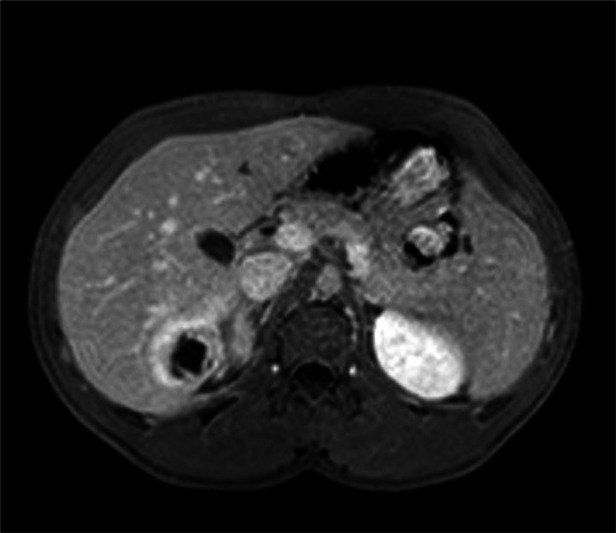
CT scan: Second collection located caudally within the same segment (S6).
